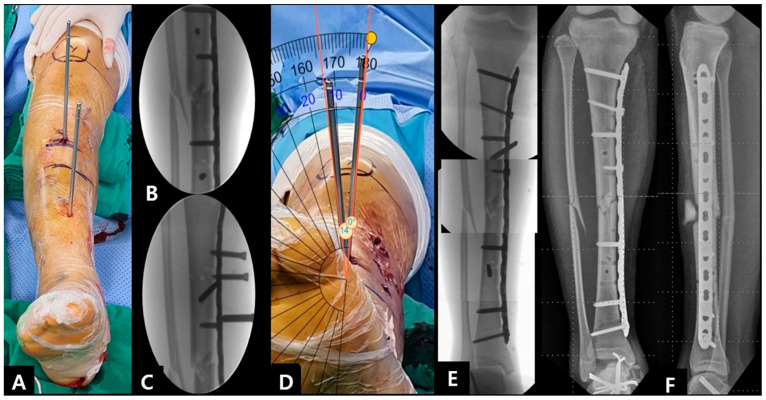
(A,B) Parallel Schanz pins placed in the proximal and distal tibia; (C) screws were removed at the proximal segment; (D) external rotation of the distal segment and the smartphone application measured about 14° correction; (E) screws were fixed at the proximal segment; (F) postoperative radiographs showing the improved alignment after revision procedure.
